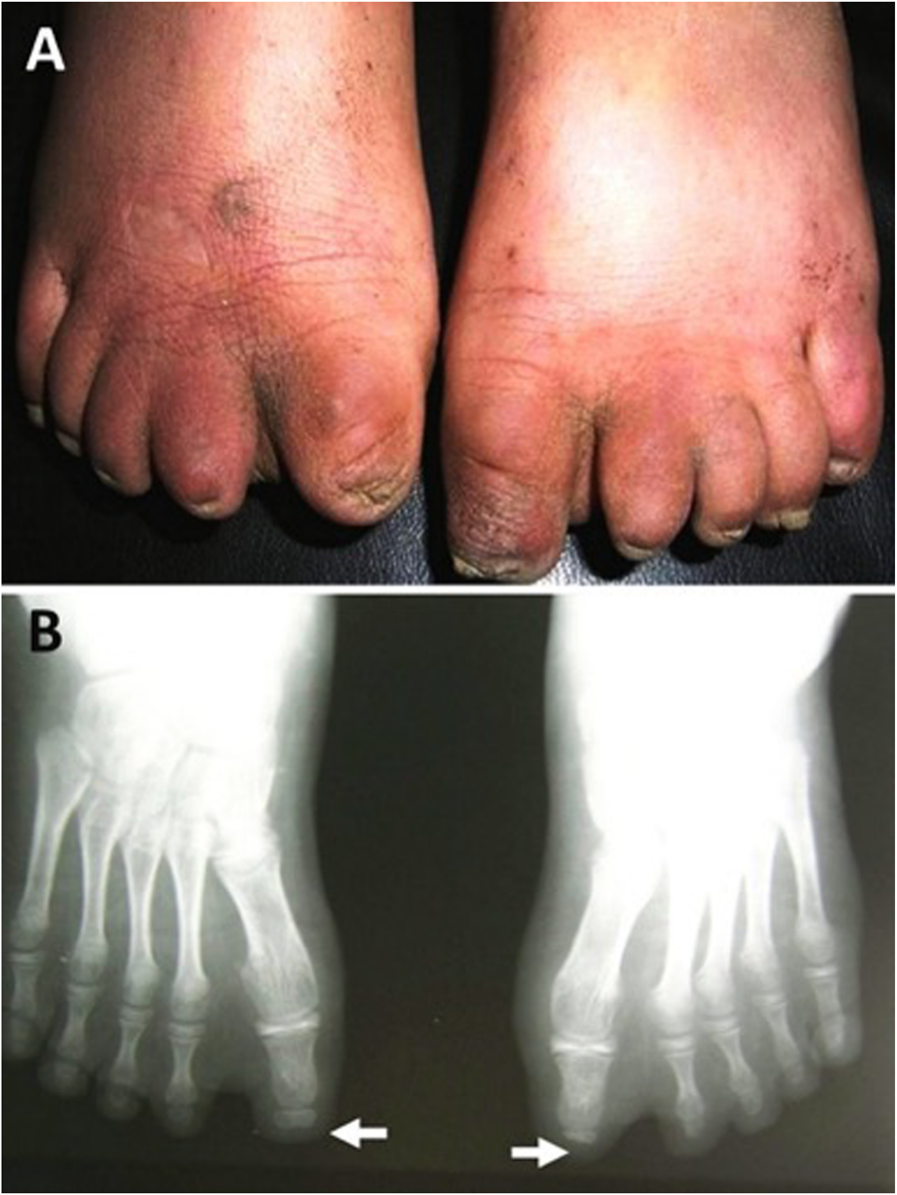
Bilateral shortening of terminal phalanges of toes (a) Foot X-ray shows hypoplasia and/or complete absence of terminal phalanges (b) [2]
